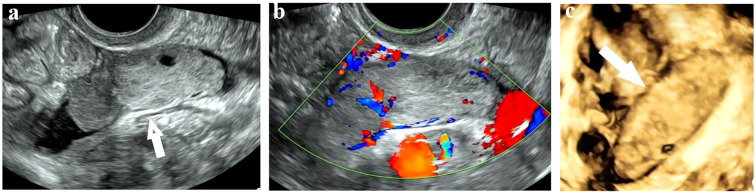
Sonographic images of Fallopian tube carcinosarcoma. (a) A solid mass with a clear boundary and regular shape in the left adnexal area. (b) Branching blood flow signals in the mass on Color Doppler mode. (c) A sausage-shaped mass detected on three-dimensional mode.
